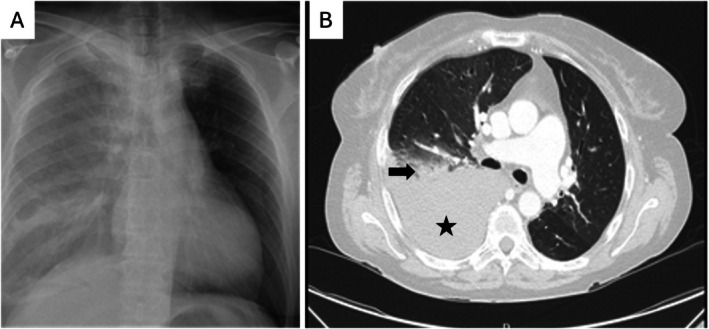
(A) Chest X‐ray showing a large right‐sided pleural effusion. (B) Chest CT scan demonstrating consolidation in the lower right lung (black arrow) and a concomitant hematoma (black star).
